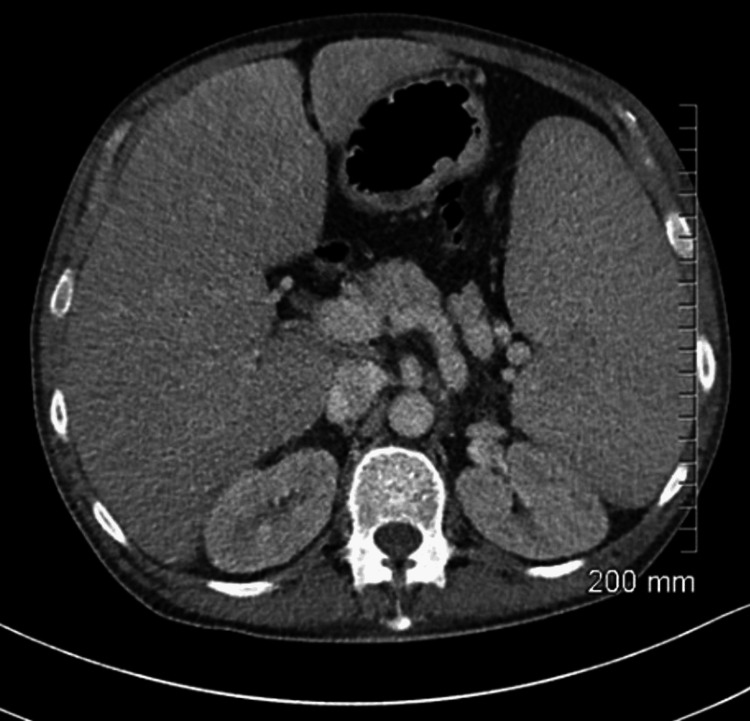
CT abdomen showing hepatosplenomegaly
